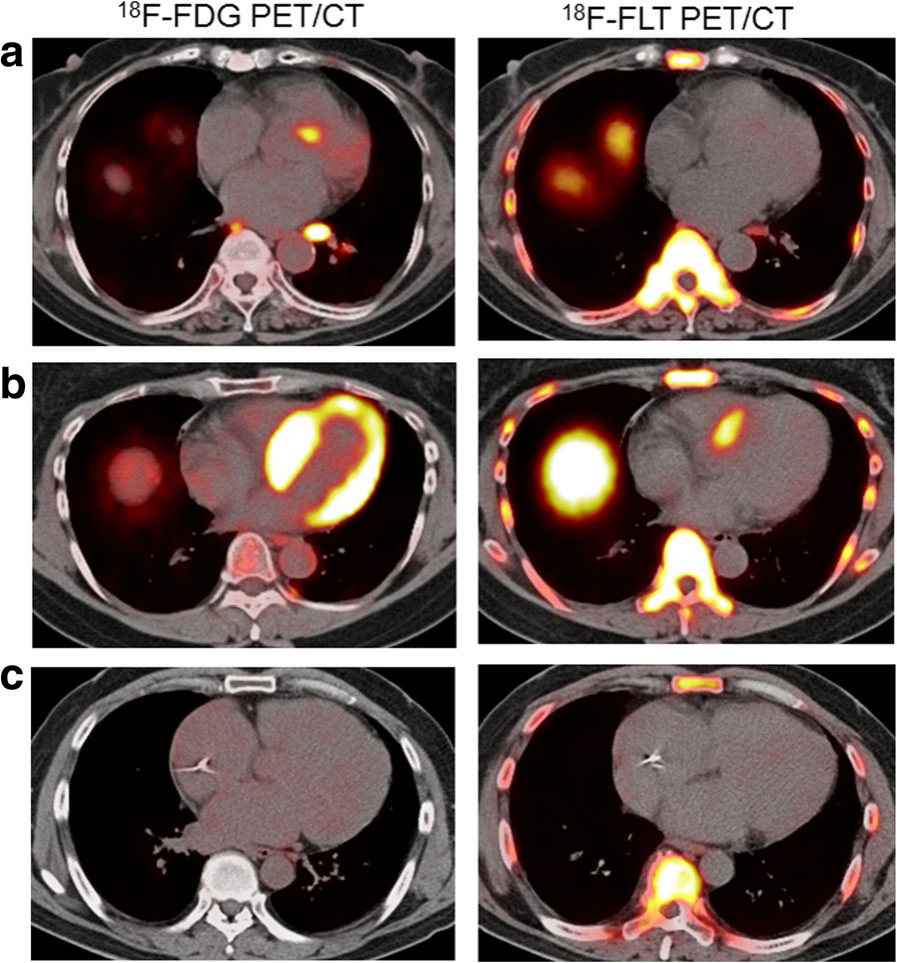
The three different patterns of cardiac uptake on transverse ^18^F-FDG and ^18^F-FLT PET/CT fusion images at cardiac level include focal ^18^F-FDG uptake in the basal anteroseptal myocardium, with no corresponding uptake of ^18^F-FLT (patient 8 in Table 1) (a), diffuse ^18^F-FDG uptake and focal ^18^F-FLT uptake in the upper basal anteroseptal myocardium (patient 11 in Table 1) (b), and no ^18^F-FDG and ^18^F-FLT uptake in the myocardium (patient 14 in Table 1) (c)
